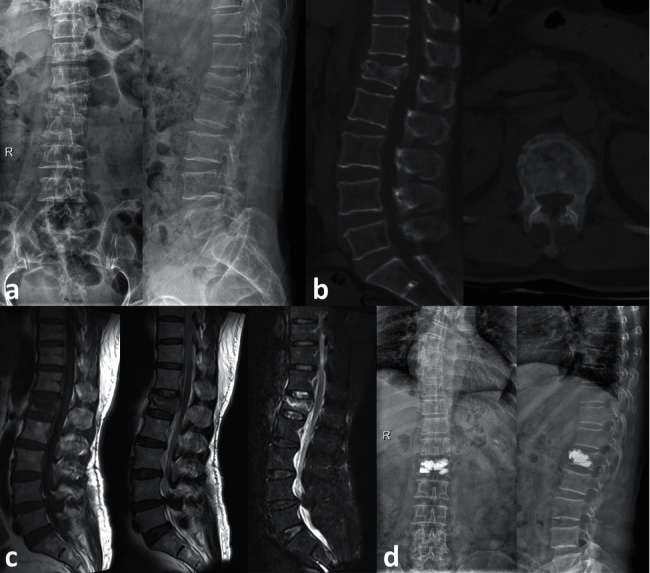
A 62-year-old female with an L1 OVCF who underwent PKP. (a) X-rays before PKP. The compression fracture of L1 can be observed. (b) CT before PKP. (c) MRI before PKP, including T1, T2, and STIR. (d) X-rays 1 day after surgery.
